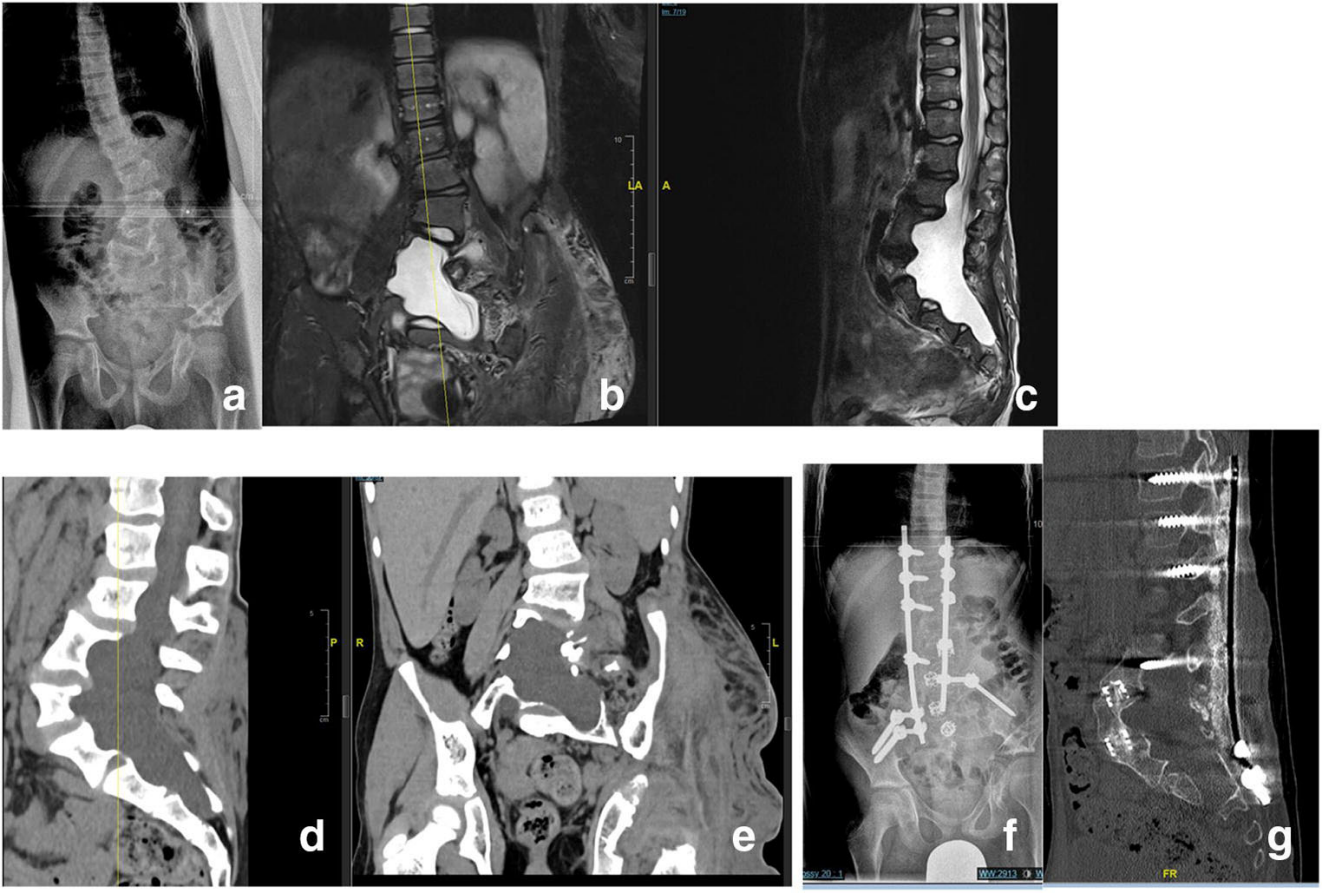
Dystrophic lumbar scoliosis (a–g) in a 13-year-old NF-1 patient (a). Notice the severe dural ectasia visible on MRI (b, c) as well as the severe bone erosion resulting in a poor bone stock visible on the preoperative CT scan (d, e).Plain X-ray and noncontrast CT scan after combined anterior and posterior spinal fusion with anterior mesh cages and “off-label” rhBMP-2 showing good correction and stable fusion on latest follow-up (f, g)
